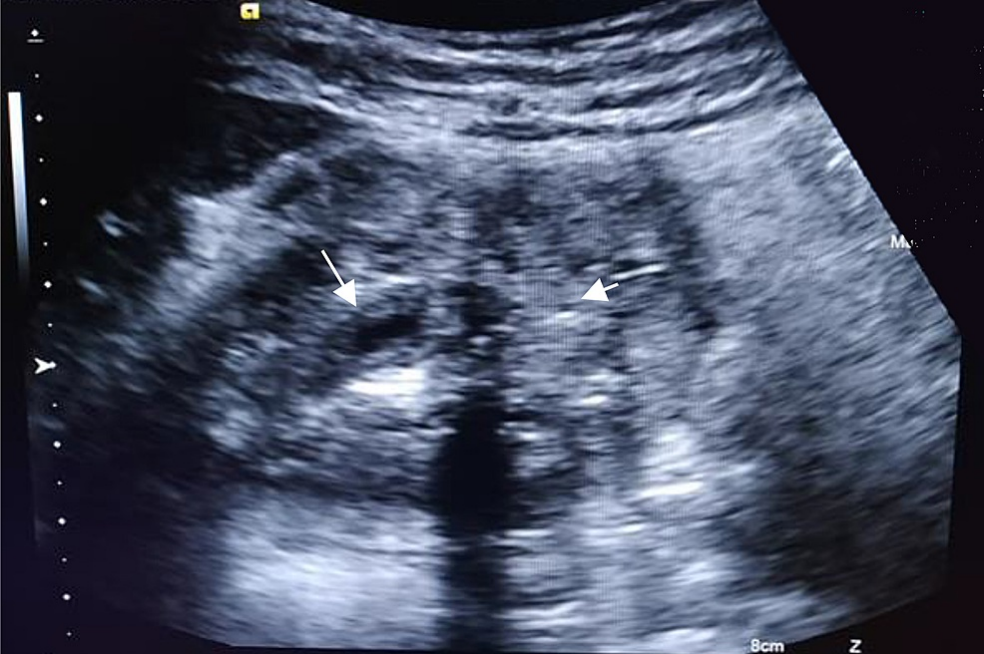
Ultrasonographic longitudinal gallbladder. Diffuse heterogenous wall thickening (short arrow). Note the regular mucosal layer (long arrow) with a small-appearing lumen with evidence of stones.
